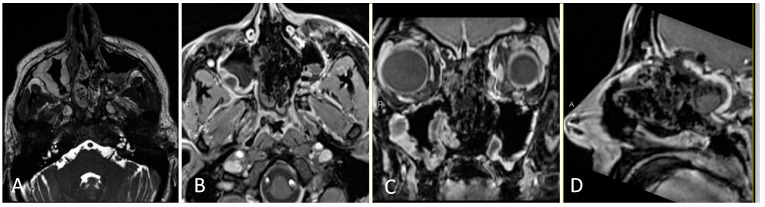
Postoperative imaging demonstrating gross total resection of a sinonasal squamous cell carcinoma. (A) Axial T2 high-resolution constructive interference steady state (CISS) T2-weighted MRI. (B) Axial T1 post-contrast MRI. (C) Coronal T1 post-contrast MRI. (D) Sagittal T1 post-contrast MRI.
